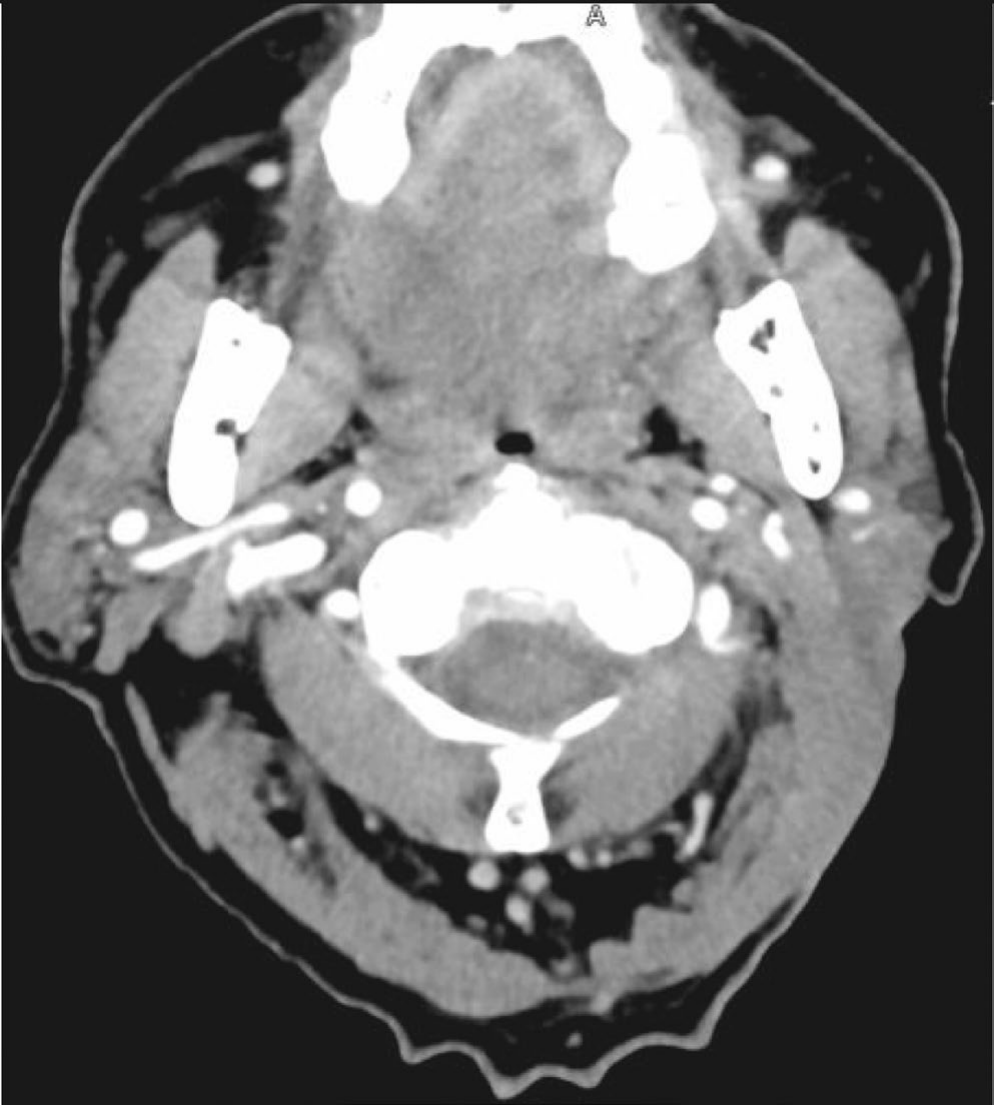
CT neck at 12 months post initiation of treatment highlighting complete radiological response to treatment.
